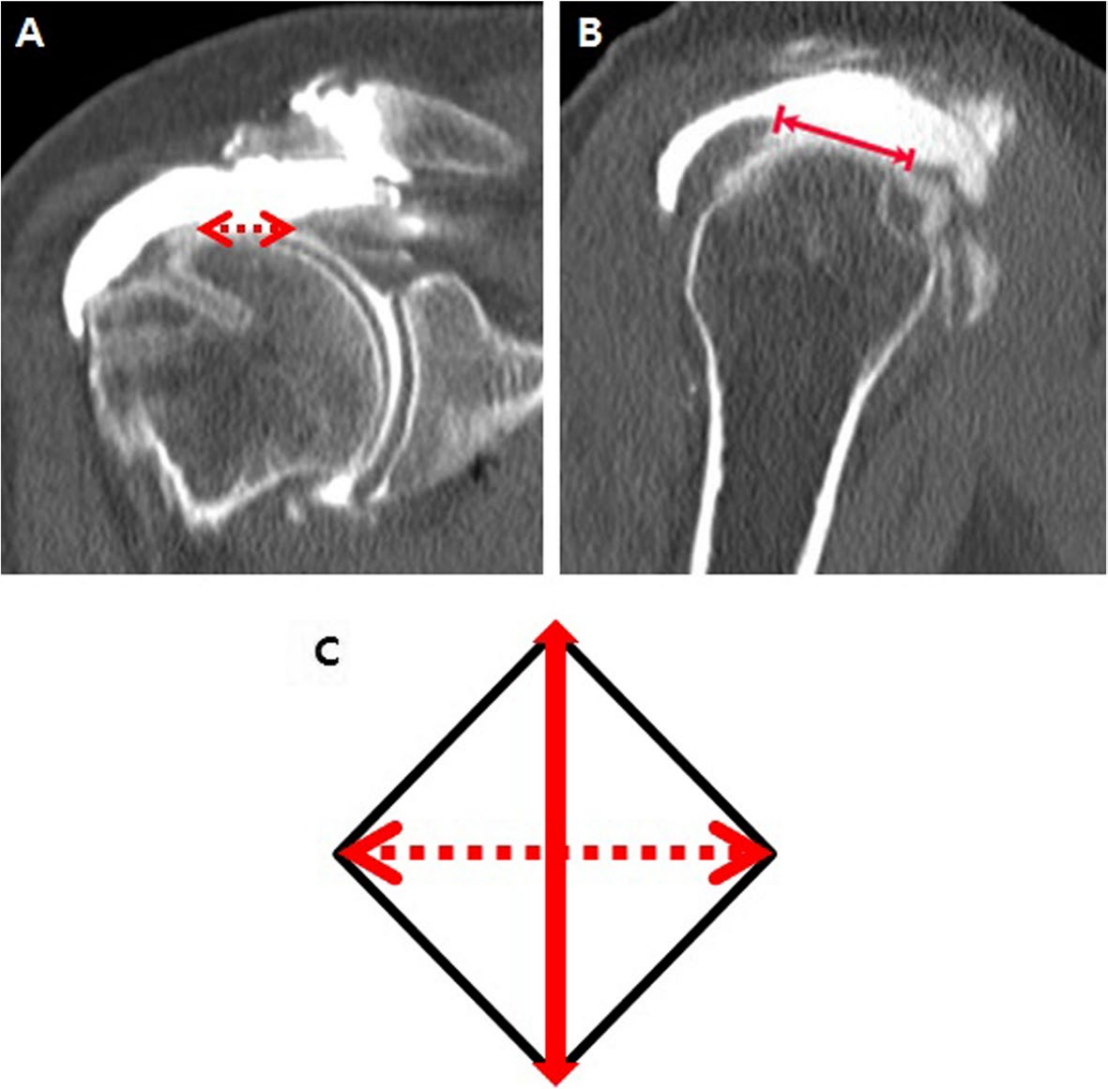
The maximum mediolateral (a) and anteroposterior (b) diameters of contrast leakage in the coronal and sagittal plane of CT arthrography were measured, respectively. c The cross-sectional area of the retorn site was calculated as 1/2 the product of the two diameters, such as the area of the rhombus
